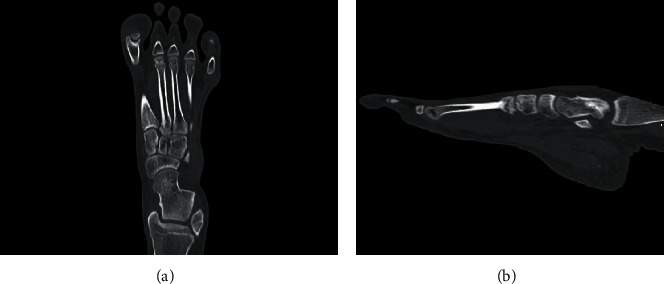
Preoperative CT demonstrated a fracture line at the base of the second metatarsal and sclerotic change around the fracture site on (a) coronal and (b) sagittal views.
